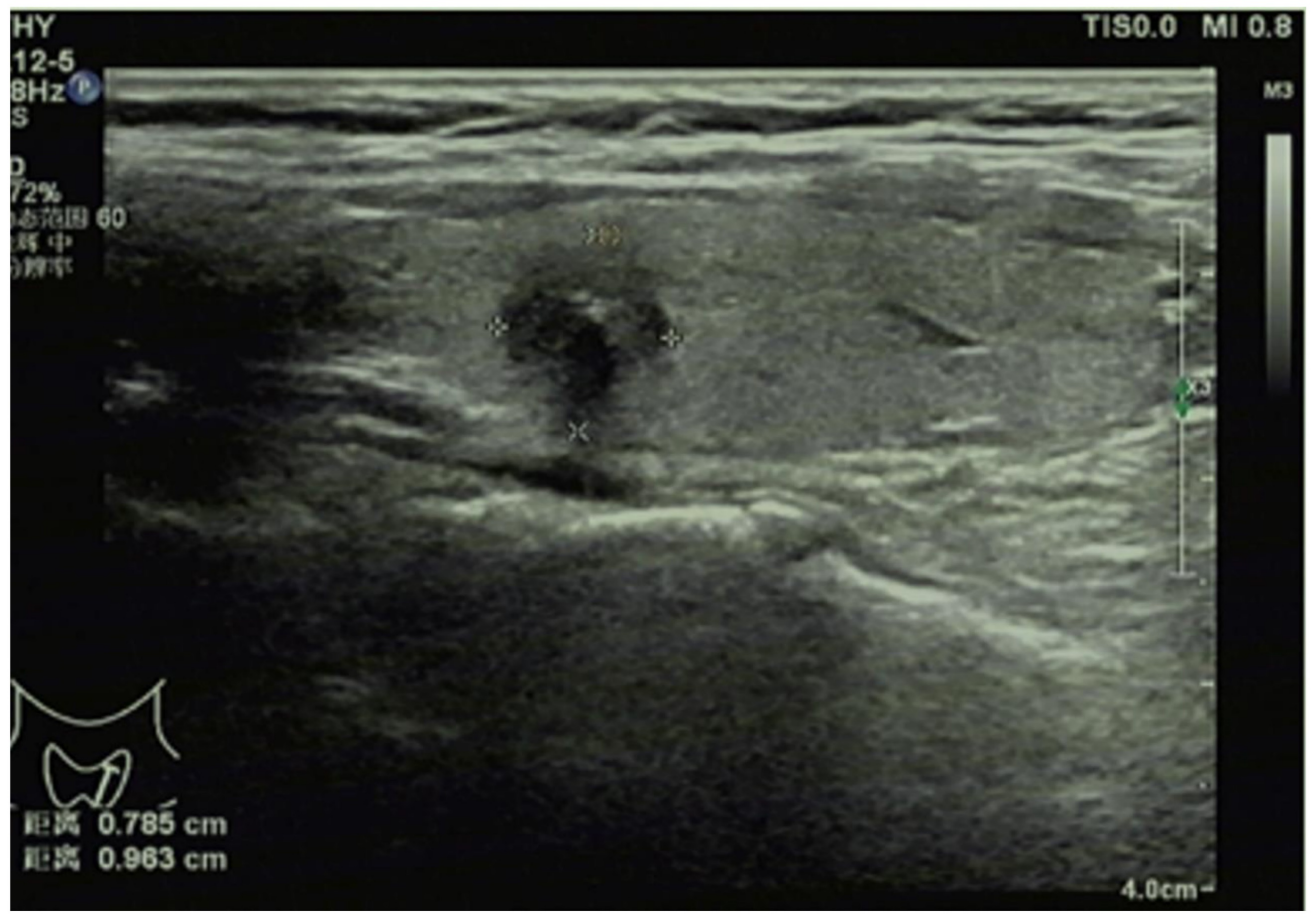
Conventional ultrasonography for papillary thyroid carcinoma.
